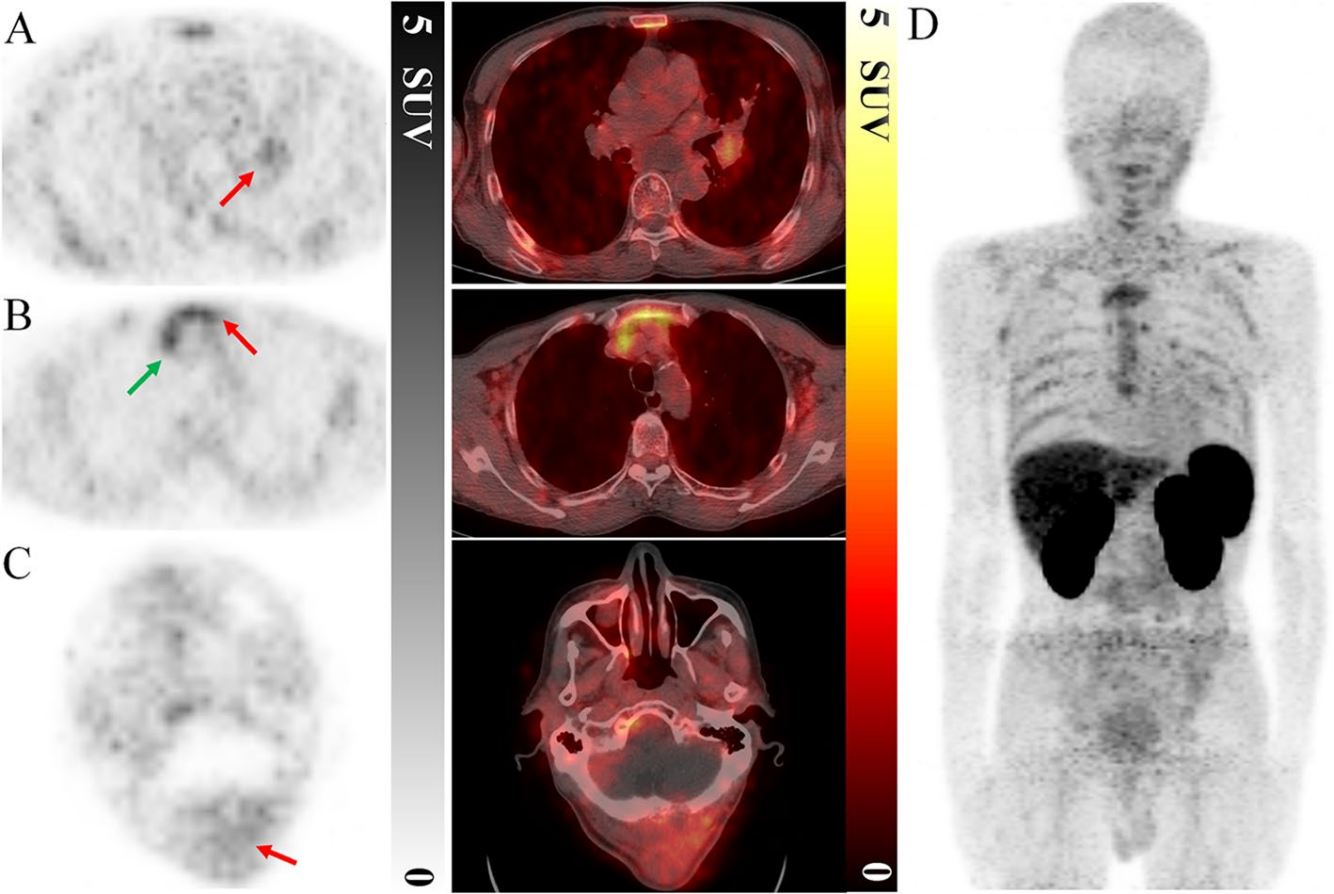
^68^Ga-NODAGA-NM-01 PET/CT of patient 2. Mild uptake in the (A) left hilum primary lesion with SUV_max_ of 1.7, while heterogeneous uptake in the (B) mediastinal lymph node with SUV_max_ of 2.4 (green arrow), sternum metastasis with SUV_max_ of 2.8 (red arrow), and (C) occipital metastasis with SUV_max_ of 1.5, respectively. D Representative maximum-intensity-projection image of patient 2
